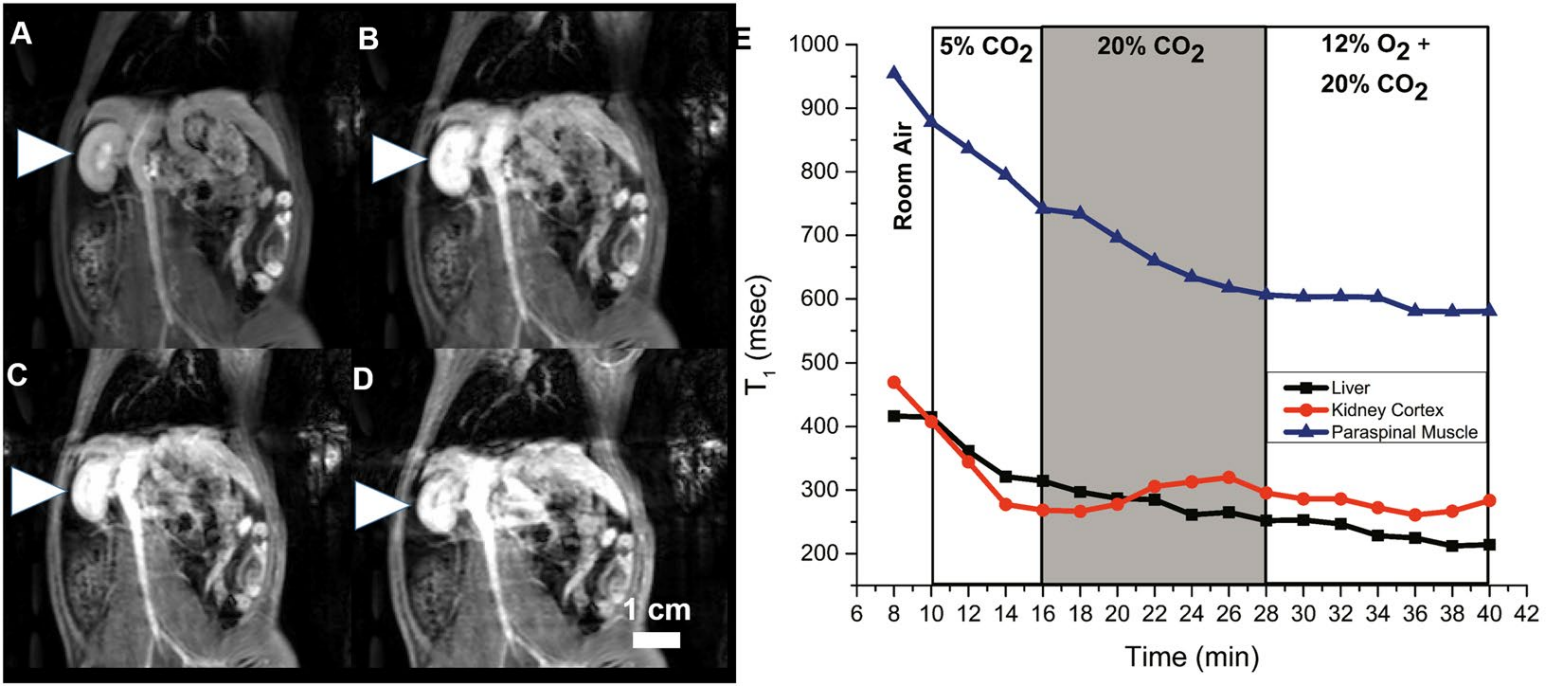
Dynamic MR imaging of blunting effect of mild hypercapnia-mediated vasodilatation on subsequent vasoconstriction capacity. The kidney is shown on *T*
